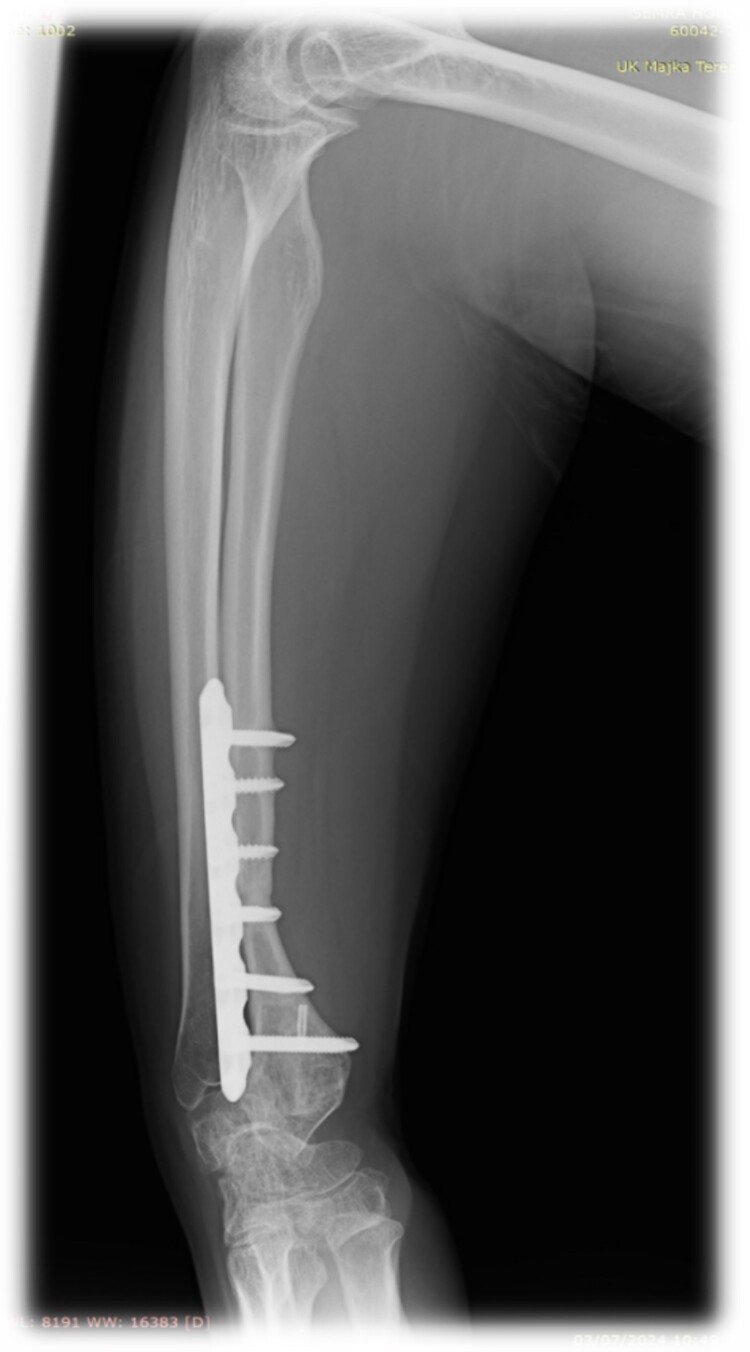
Follow-up X-ray of the right forearm 2 years after the surgery in profile plane
